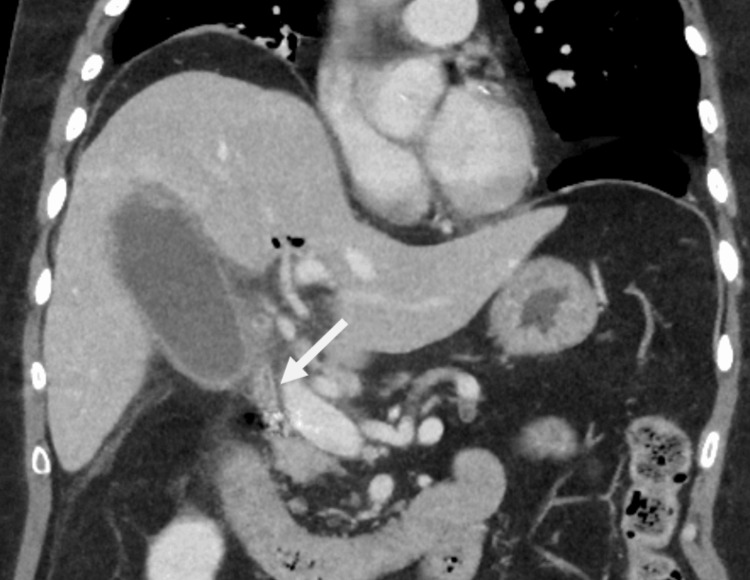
Coronal slice of a CT abdomen and pelvis with contrast (portovenous phase). The image shows a non-dilated CBD (white arrow). CBD: common bile duct
